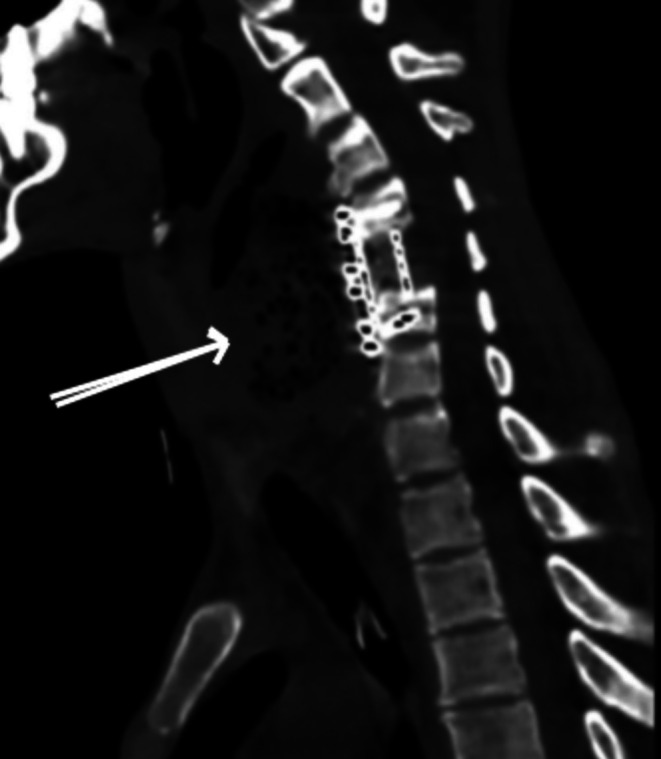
Cervical computed tomography demonstrating retropharyngeal air adjacent to the anterior cervical plate, suggestive of esophageal perforation.
